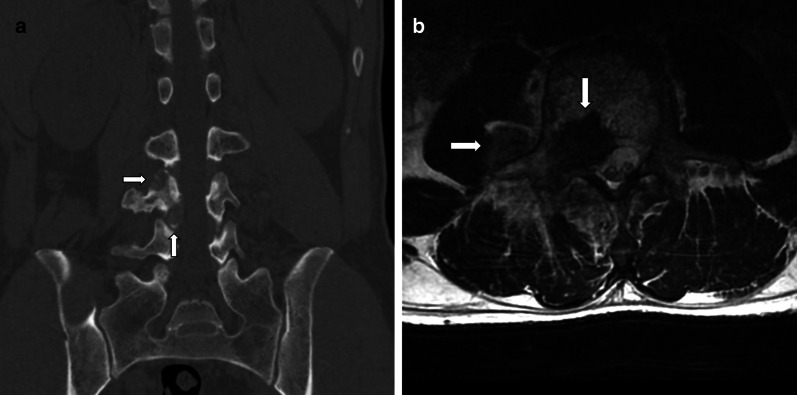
A 44-year-old man with multifocal lesions on the right accessory of L4 that involved to the vertebral body. CT shows two obvious niduses in the right accessory (a). An isointense signal nidus and a slight hyperintense signal nidus can be shown on T2WI (b)
